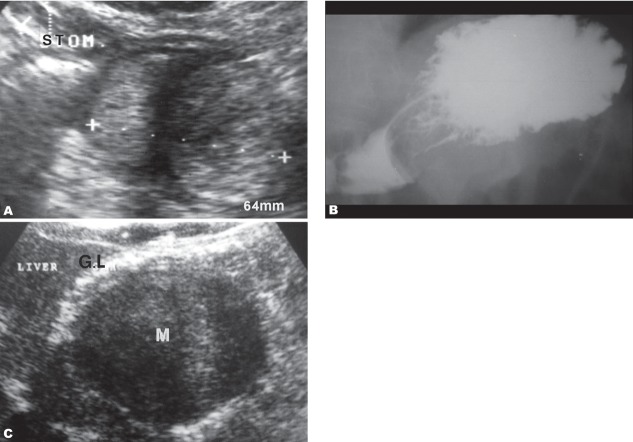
Abdominal ultrasound (A) and barium meal (B) images in a 47year-old lady with gastric wall leiomyoma. In another similar case (C), ultrasound image shows displacement of the gastric lumen (GL) gas by the submucosal mass lesion (M).
